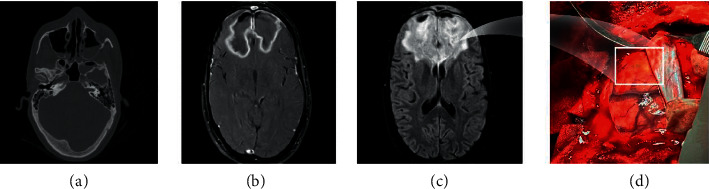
Radiologic characteristics of COVID-19-associated mucormycosis: (a) axial bone window CT scan demonstrated partial opacification of bilateral maxillary and sphenoid sinuses. (b) Axial postcontrast T1 weighted image revealed smooth ring enhancement in bilateral frontal lobes mass; (c) axial fluid-attenuated inversion recovery MRI showed a hyperintense mass containing air bubbles with perilesional vasogenic edema in bilateral frontal lobes; (d) drainage of the yellow brain abscess material using craniotomy.
